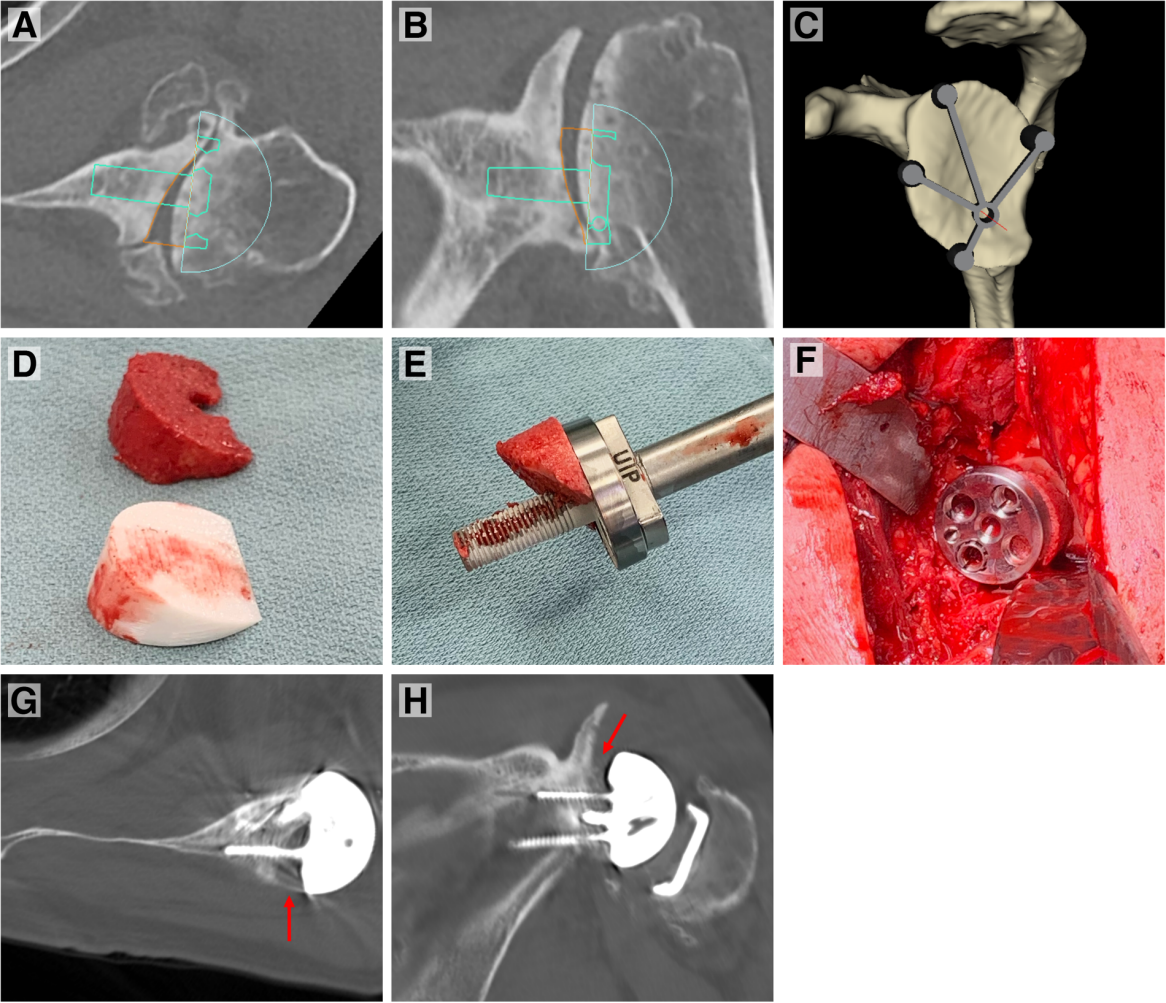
(A, B, and C) Preoperative 3D templating of the presented case revealing substantial glenoid erosion and deformity (ie, retroversion). Glenoid baseplate positioning, graft dimensions, and central pin placement is templated. (D) Comparison of graft harvested utilizing the 3D-printed cutting guide and the 3D-printed replica of the wedge deemed necessary to correct the patient's deformity. (E and D) Intraoperative pictures showing the graft inserted on the glenoid baseplate and impacted onto the glenoid in appropriate orientation. (G and H) Postoperative axial and coronal CT images demonstrating correction of glenoid version and inclination and position of the bone graft. (Arrows pointing to the bone graft positioned under the glenoid baseplate). Abbreviations: 3D, three-dimensional; CT, computed tomography.
